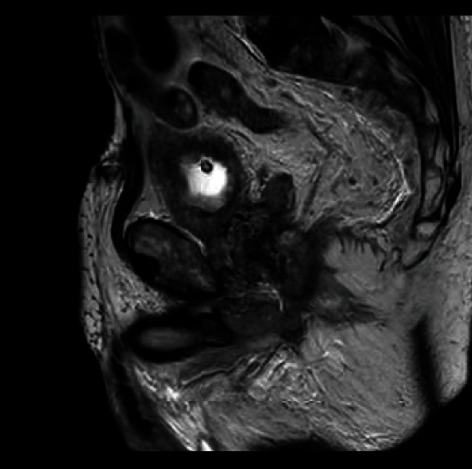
Sagittal MRI of periprostatic collection.
